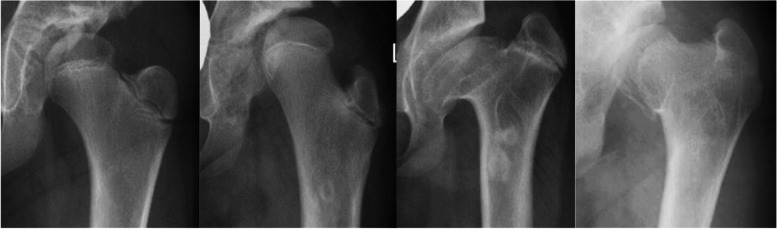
Radiographs representing Grade I, Grade II, Grade III, and Grade IV (from left to right) of the Bucholz-Ogden classification for osteonecrosis secondary to DDH
